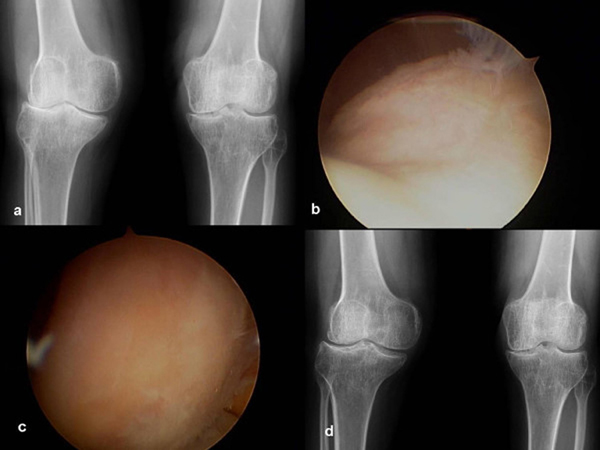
(a) Pre-operatively, both knee standing anteroposterior radiographs show lateral joint space narrowing and a 13° valgus deformity. Arthroscopic findings of the (b) trochlea and (c) lateral condyle show severe arthritic change. (d) Both knee standing anteroposterior radiographs obtained 12 months after the autologous chondrocyte implantation surgery show widening of the lateral joint space and improvement in the valgus deformity to 7°.
